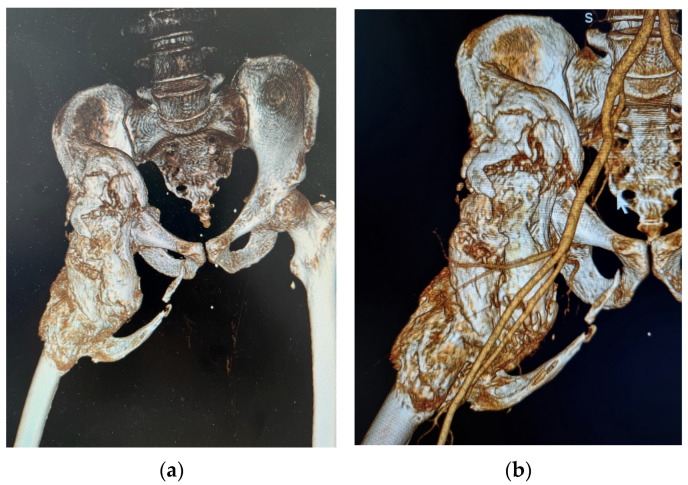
3D reconstruction of preoperative CT imaging in RadiAnt DICOM for the patient included in the study: (a) native window; (b) arterial window.
